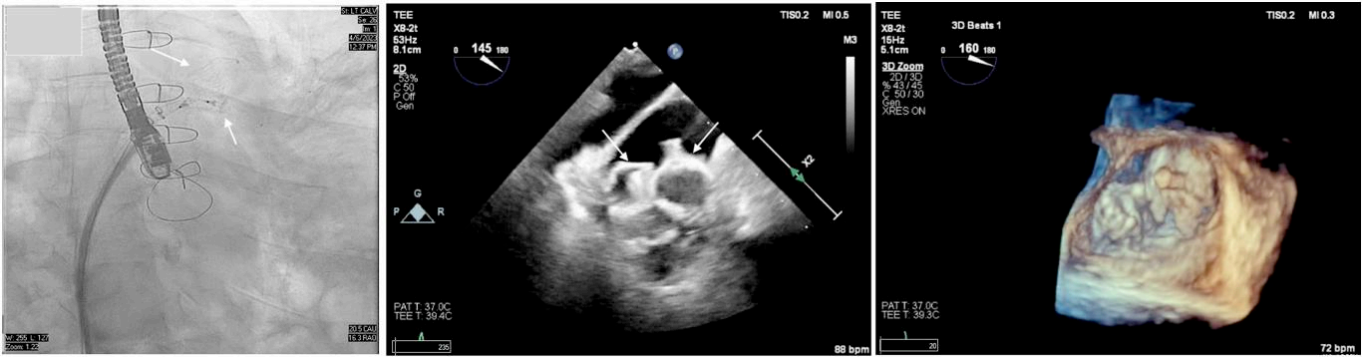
Transoesophageal echocardiogram with three-dimensional imaging and cine angiography showing successfully deployed two WATCHMAN FLX devices.
